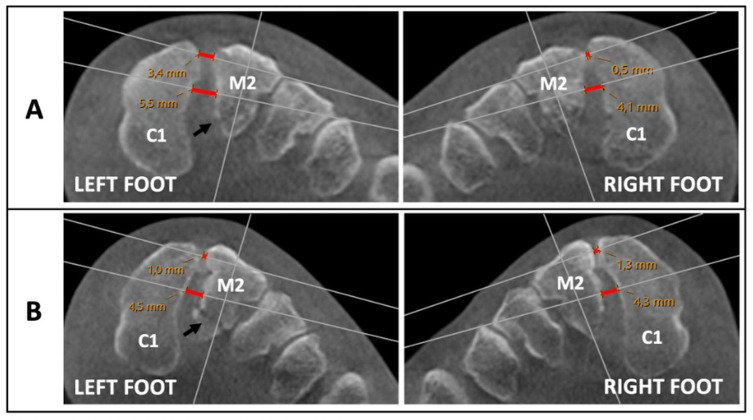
Weightbearing computed tomography assessment in 2 patients (A and B) with a similar-looking, subtle Lisfranc injuries to the left foot. Fracture is indicated with a black arrow. The corresponding measurements of the dorsal Lisfranc ligament (DLL) and the interosseous Lisfranc ligament (ILL) are highlighted in red along the measurement axis. Patient A was classified as unstable, with a difference score of 4.3 mm (left: DLL 3.4 mm, ILL 5.5 mm; right: DLL 0.5 mm, ILL 4.1 mm). Notably, the side-by-side difference of the ILL was only 1.4 mm. Patient B was classified as stable, with a difference score of 0.1 mm (Left: DLL 1.0 mm, ILL 4.5 mm; Right: DLL 1.3 mm, ILL 4.3 mm). C1 = medial cuneiform, M2 = second metatarsal. [See online article for color figure.]
